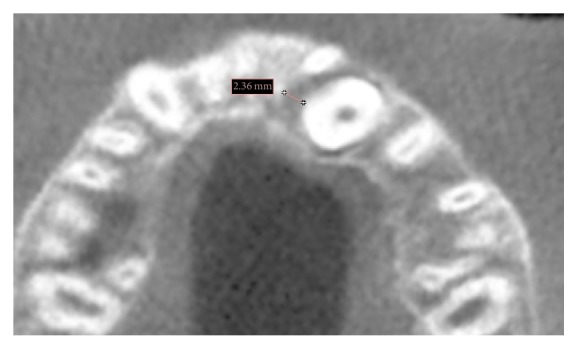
Width measurement of the follicle in the axial CBCT image.
